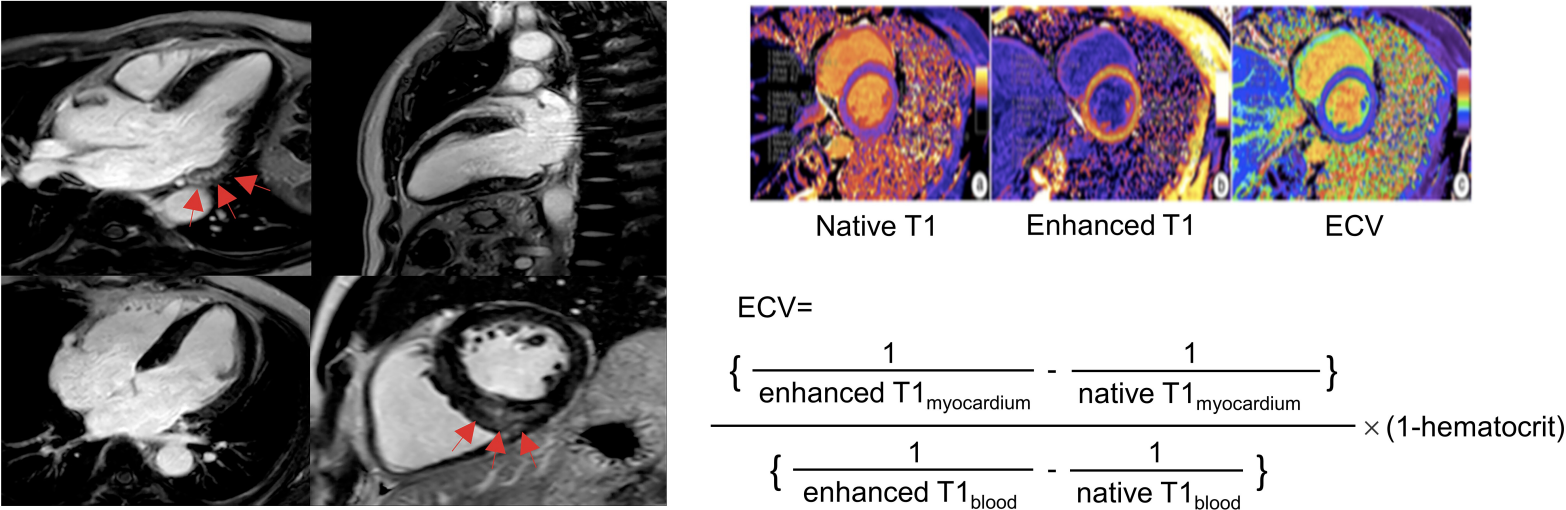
Late gadolinium enhancement (left) and extracellular volume fraction (right) analyses in primary aldosteronism. The cardiac fibrosis of late gadolinium enhancement on the left panel was indicated by the red arrow at basal inferolateral (upper left) and inferoseptal (bottom right) segments presented by focal high signal intensity in mid-layer myocardium on the left panel. Reproduced from ‘Chen YL, et al. Non-invasive left ventricular pressure-strain loop study on cardiac fibrosis in primary aldosteronism: A comparative study with cardiac magnetic resonance imaging. *Hypertension Research*. 2024. Springer Nature.’ (20).
